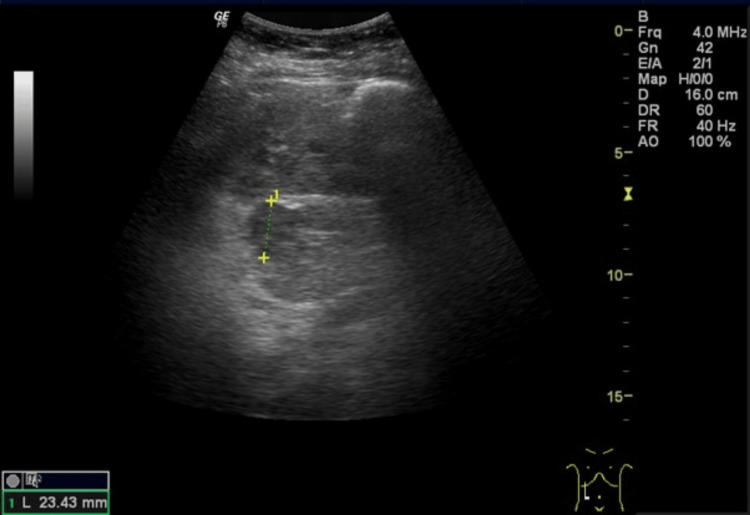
Renal ultrasound revealing the presence of a right pararenal mass measuring 23.43 mm.
